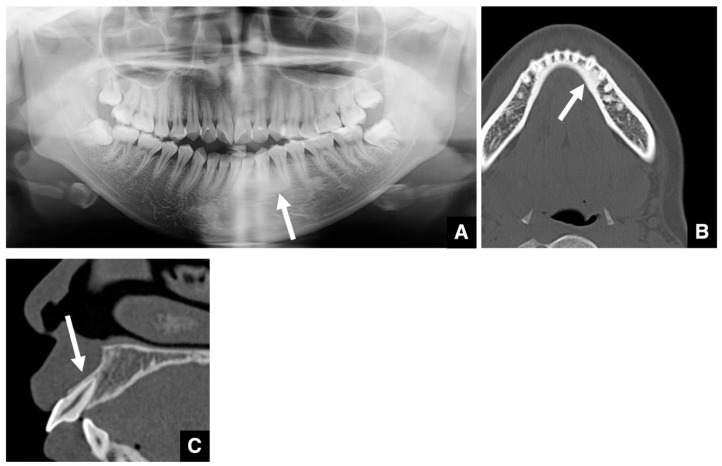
Evaluation of the relationship between tooth roots and surrounding bone structures in planning for orthodontic movement. (A) Panoramic radiograph of a patient with enostosis (arrow). (B) Axial bone window MDCT image (slice thickness, 3.0 mm) of the region with enostosis (arrow). The relationship between the tooth root and the enostosis should be evaluated, and root movement should be planned to avoid the lesion. (C) Sagittal bone window MDCT image (slice thickness, 2.0 mm) shows contact between the cortical bone and the tooth root (arrow). Attention should be paid to the relationship between the cortical bone and the root to avoid applying pressure on the cortical bone during tooth movement. Permission has been obtained to publish all figures.
